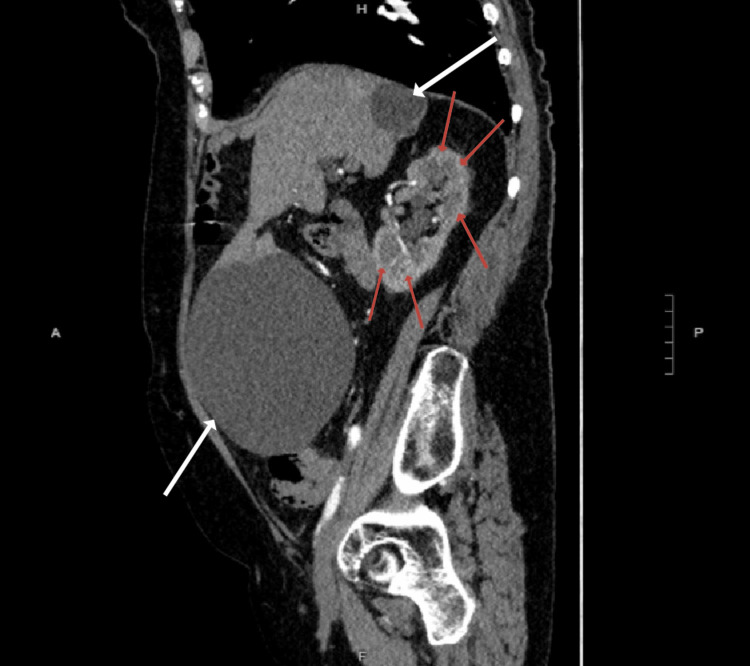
The CT image of the abdomen in the sagittal plane showing numerous renal cysts in the right kidney and large hepatic duplication cysts on the anterior and posterior right lobe of the liver. Red arrows: Renal cysts, White arrows: Hepatic duplication cysts
